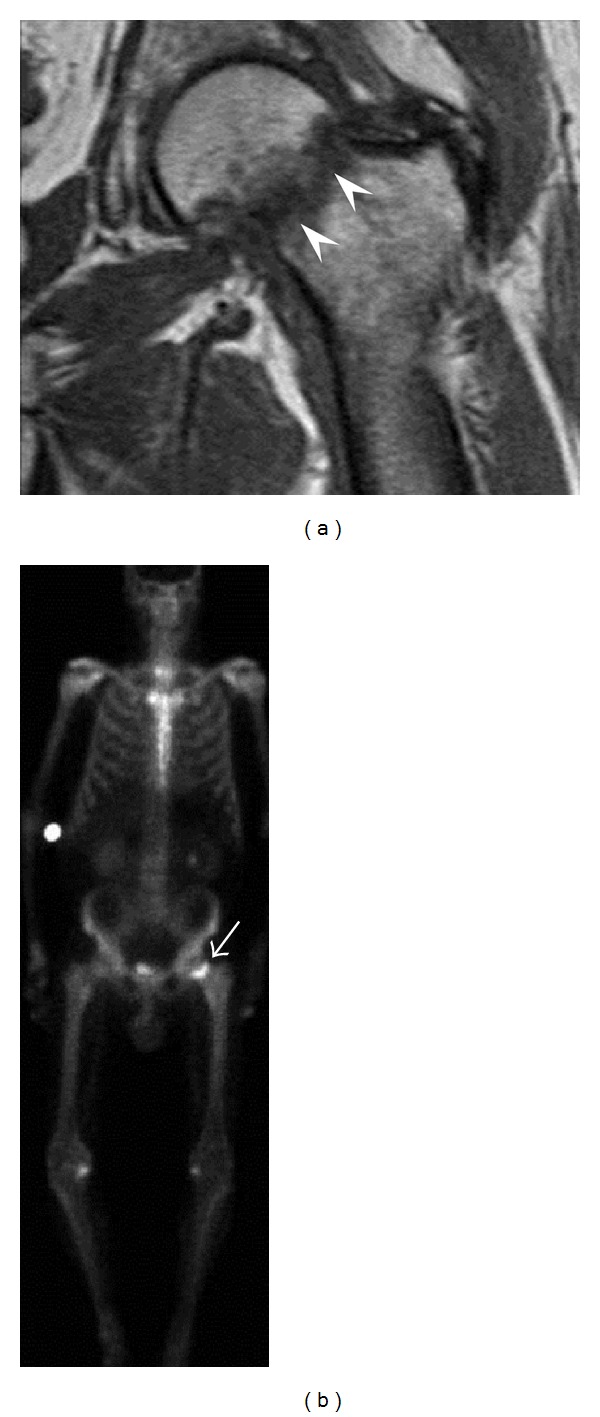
Subcapital insufficiency fracture in a 55-year-old man with a left hip pain without a history of trauma. Anteroposterior and Lauenstein view radiographs centered on the left hip do not show an obvious fracture line, but mild acetabular osteophytosis was noted consistent with hip osteoarthritis (not shown). (a) Coronal T1-weighted MRI shows a linear low-signal band through the femoral neck corresponding to a fracture line (arrowheads). (b) Bone scintigraphy shows focal uptake (arrow) corresponding to the fracture.
